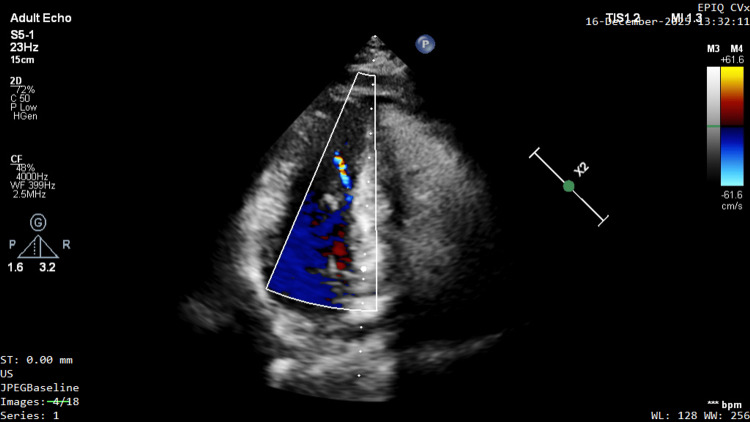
Identification of an apical jet suggesting a hidden aneurysm. Transthoracic echocardiography shows a modified apical four-chamber view with posterior angulation. Color Doppler imaging reveals an abnormal, high-velocity systolic jet originating from the left ventricular apex. The apical myocardial wall is markedly thickened (2.0 cm), raising clinical suspicion of an apical aneurysm, despite the absence of a visible aneurysmal sac in the standard four-chamber view.
